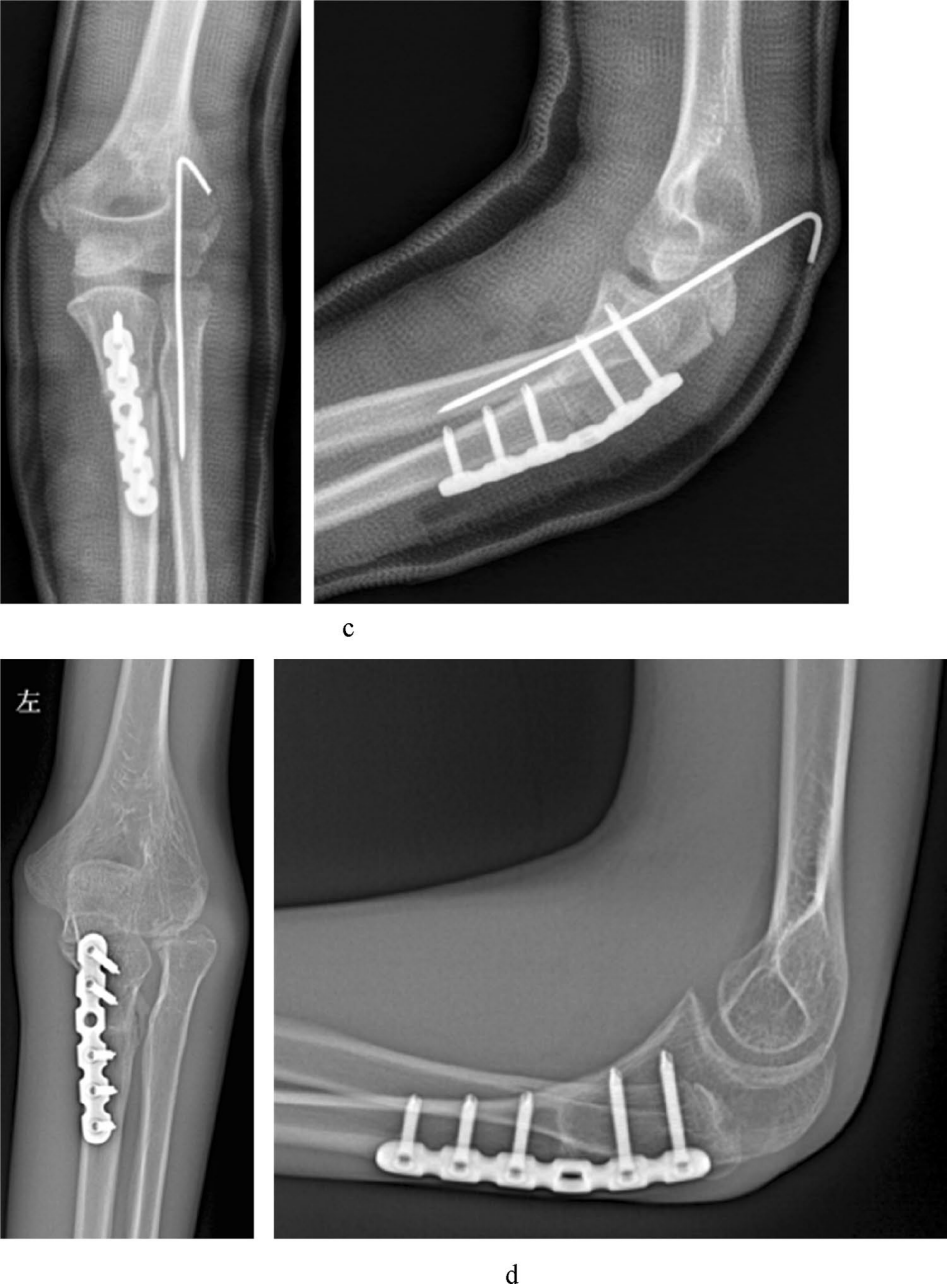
A 13 years old boy (case 12) with a missed Monteggia lesion after a 7 years interval. (a) Preoperative X-ray. The appearance of the radial head was domed. (b) Preoperative MRI. Axial T1- and T2-weighted images showed the proximal radioulnar joint was dislocated and the appearance of the radial notch (the arrow) was domed; coronal T2-weighted images showed the radial notch of the ulna was severe hyperplasia (the arrow); sagittal T2-weighted images showed the dislocation of the humeroradial joint and the domed radial head (the arrow) with dense fibrous scar tissue. (c) Open reduction and ulnar osteotomy with reconstruction of the annular ligament and a humeroradial pin was undertaken. (d) The radial head dislocated (Grade 5) due to the hyperplastic radial head incongruency with the capitellum and the elbow had osteoarthritic changes posteriorly at 3 years after operation.
